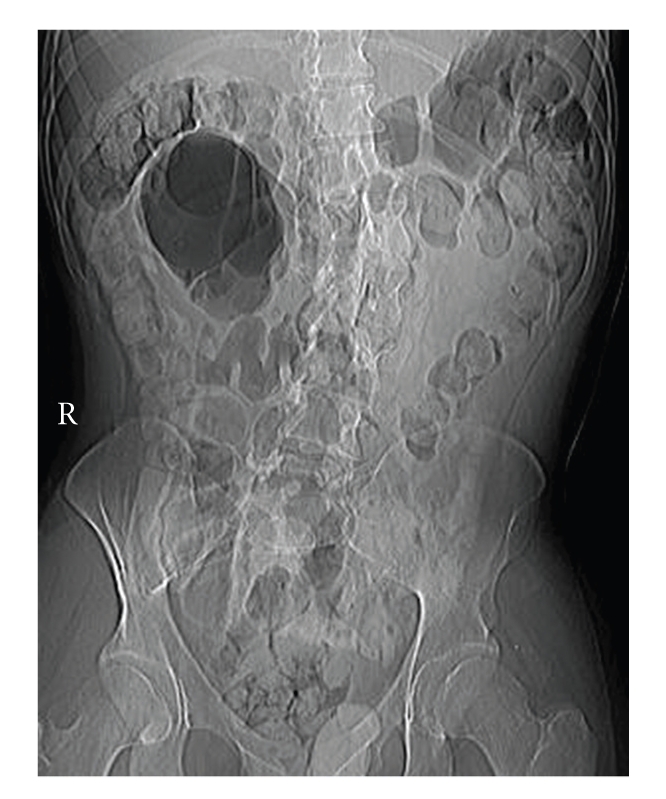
Abdominal X-ray demonstrating a cystic air-filled structure in the right upper quadrant.
